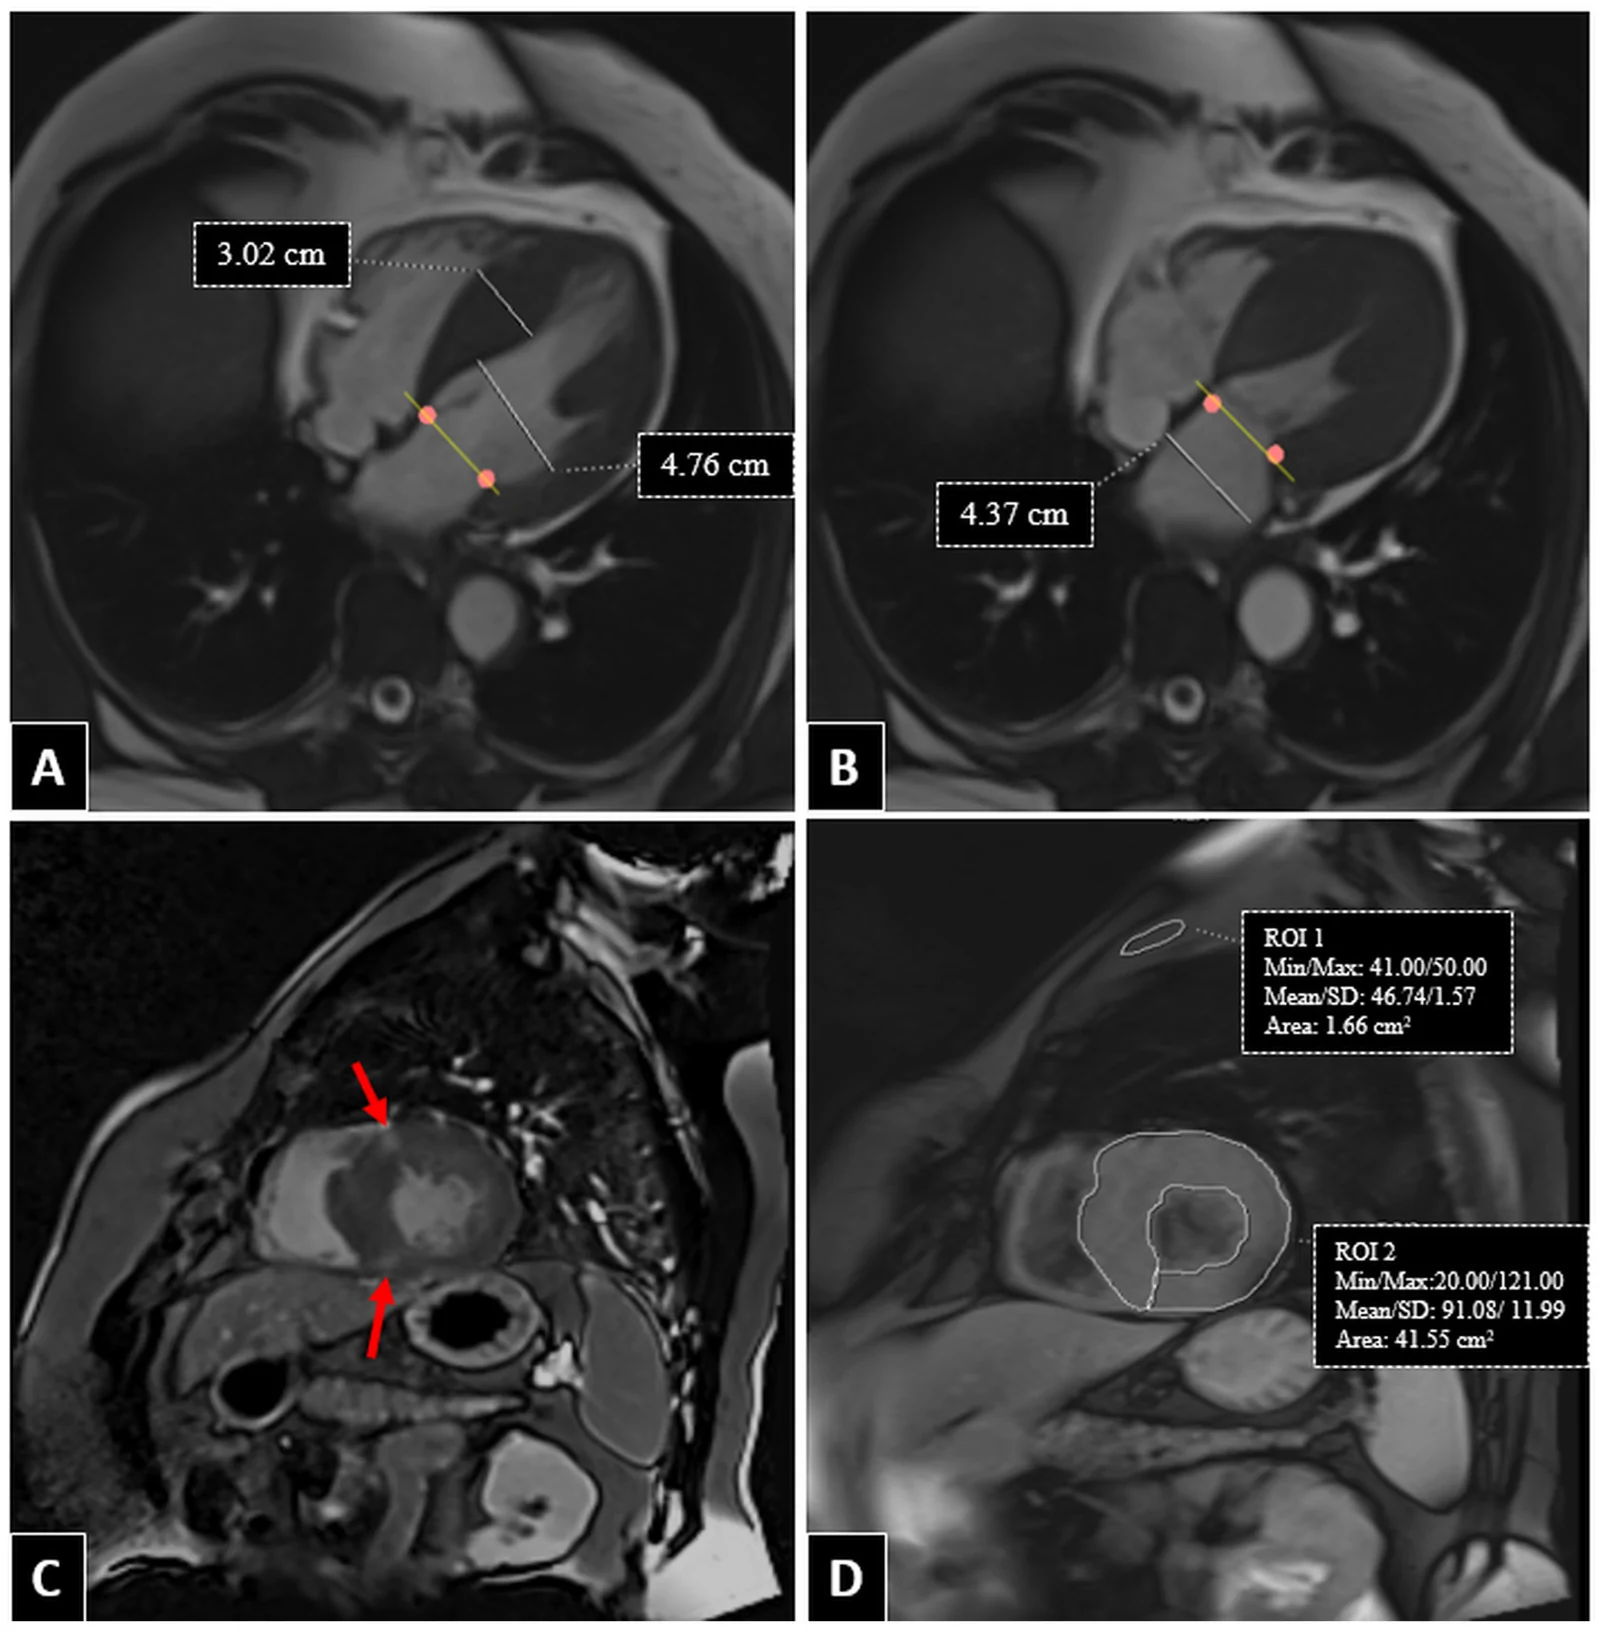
CMR images from a 40-year-old man with hypertrophic cardiomyopathy and LV systolic dysfunction (LVEF, 35%). (A) Four-chamber end-diastolic cine image showing marked interventricular septal thickening, measuring 3.02 cm (30.2 mm; short white line), and an LV cavity diameter of 4.76 cm (47.6 mm; long white line). The yellow line connecting the red dots indicates the level of the mitral valves. (B) Four-chamber end-systolic cine image showing an LA diameter of 4.37 cm (43.7 mm; white line). The yellow line connecting the red dots indicates the level of the mitral valves. (C) LGE image showing patchy nodular enhancement in the interventricular septum and at the right ventricular insertion points (red arrows). (D) Measurement of myocardial and serratus anterior muscle signal intensities on a T2-weighted TIRM image.
